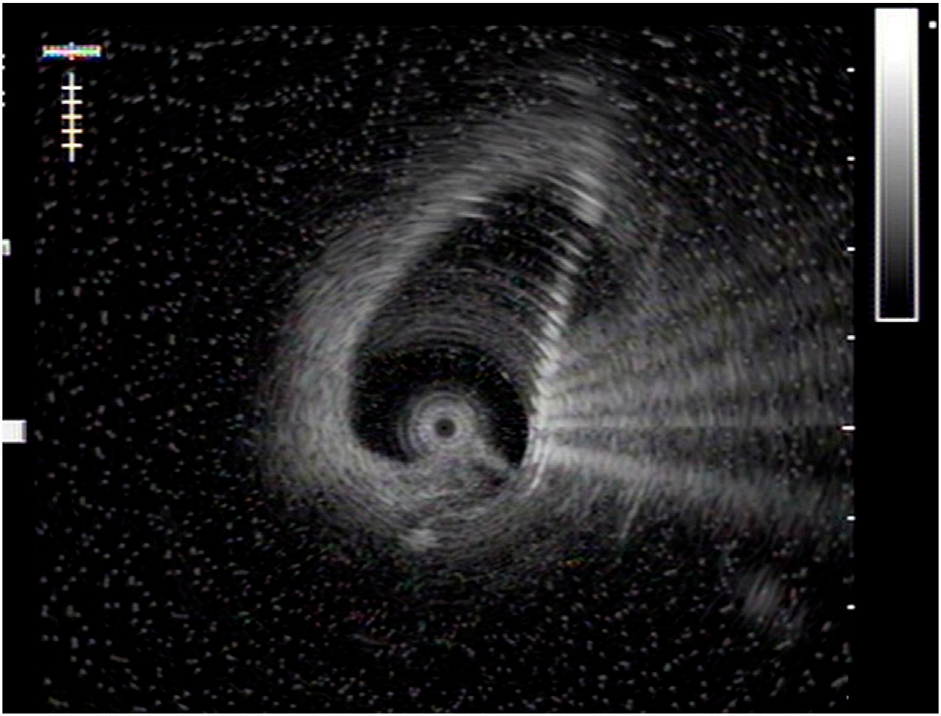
Ultrasonic image of ridge.
